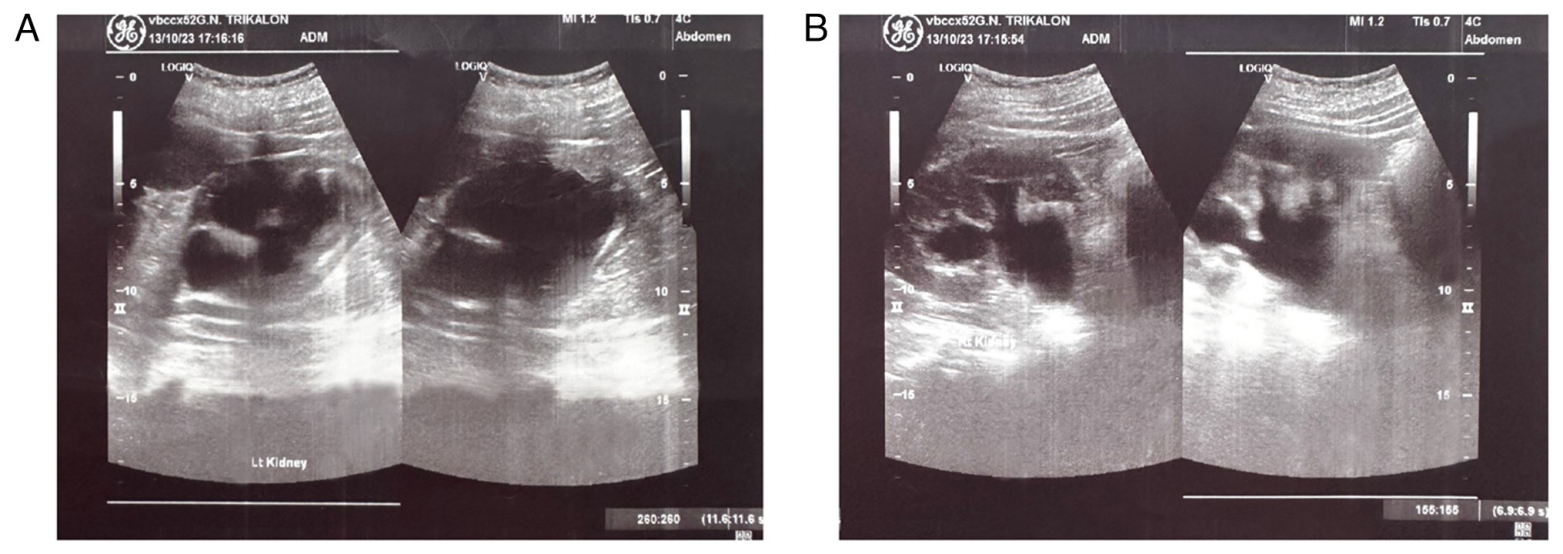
Preoperative ultrasound image of the kidneys revealing bilateral dilatation of the pelvicalyceal system and the upper ureters: (A) Right kidney, (B) left kidney.
